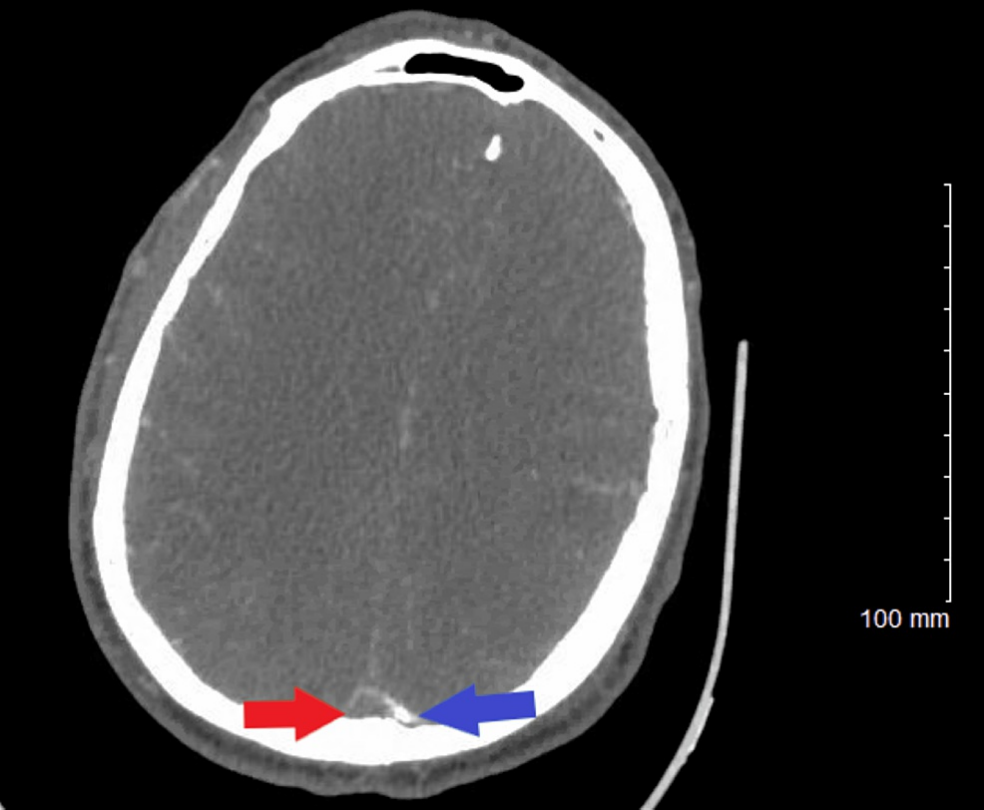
Computed tomography angiography of the head (axial view). The red arrow shows superior sagittal sinus thrombosis, and the blue arrow shows decreasing vessel patency.
